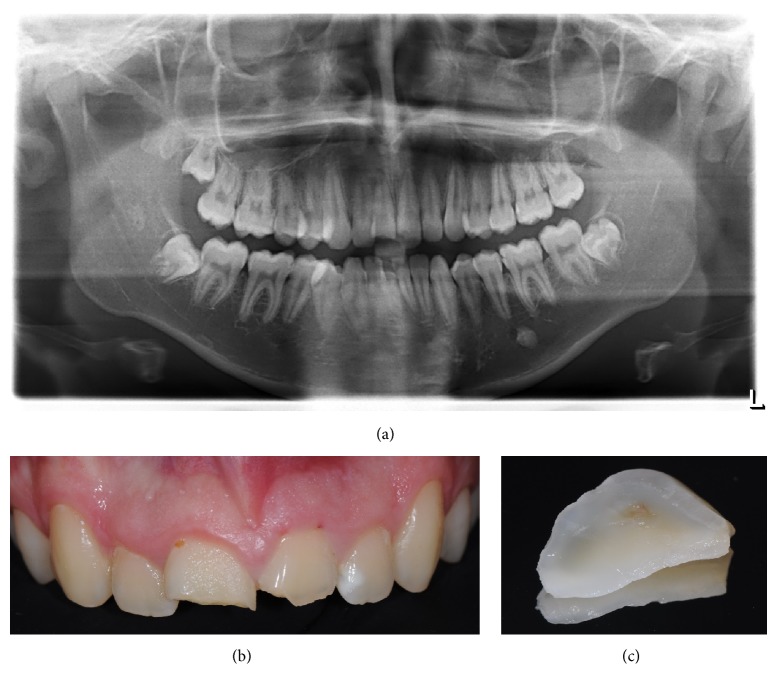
(a) Initial orthopantomography. (b) Preoperative clinical view. (c) Tooth fragment.
